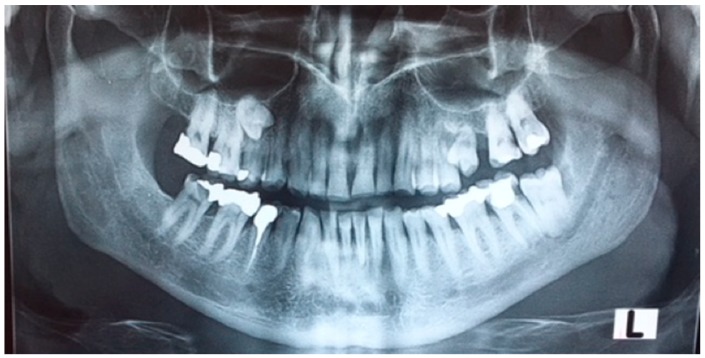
As seen in the panoramic radiograph of the father, the paramolars are located bilaterally in the maxilla. The right one is impacted, while the left one is partially erupted after the extraction of the first left molar.
